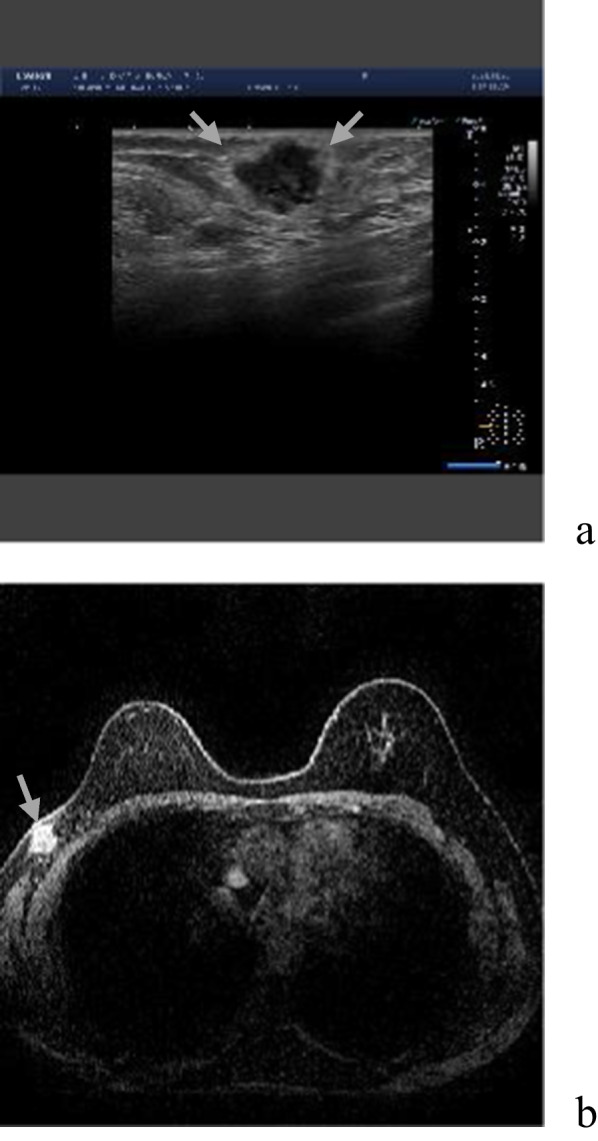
Ultrasonography and magnetic resonance imaging of the tumor. Ultrasonography scans revealed a 21.3-mm tumor in breast (a). Magnetic resonance imaging revealed 16 mm irregular geometries close to the skin (b)
